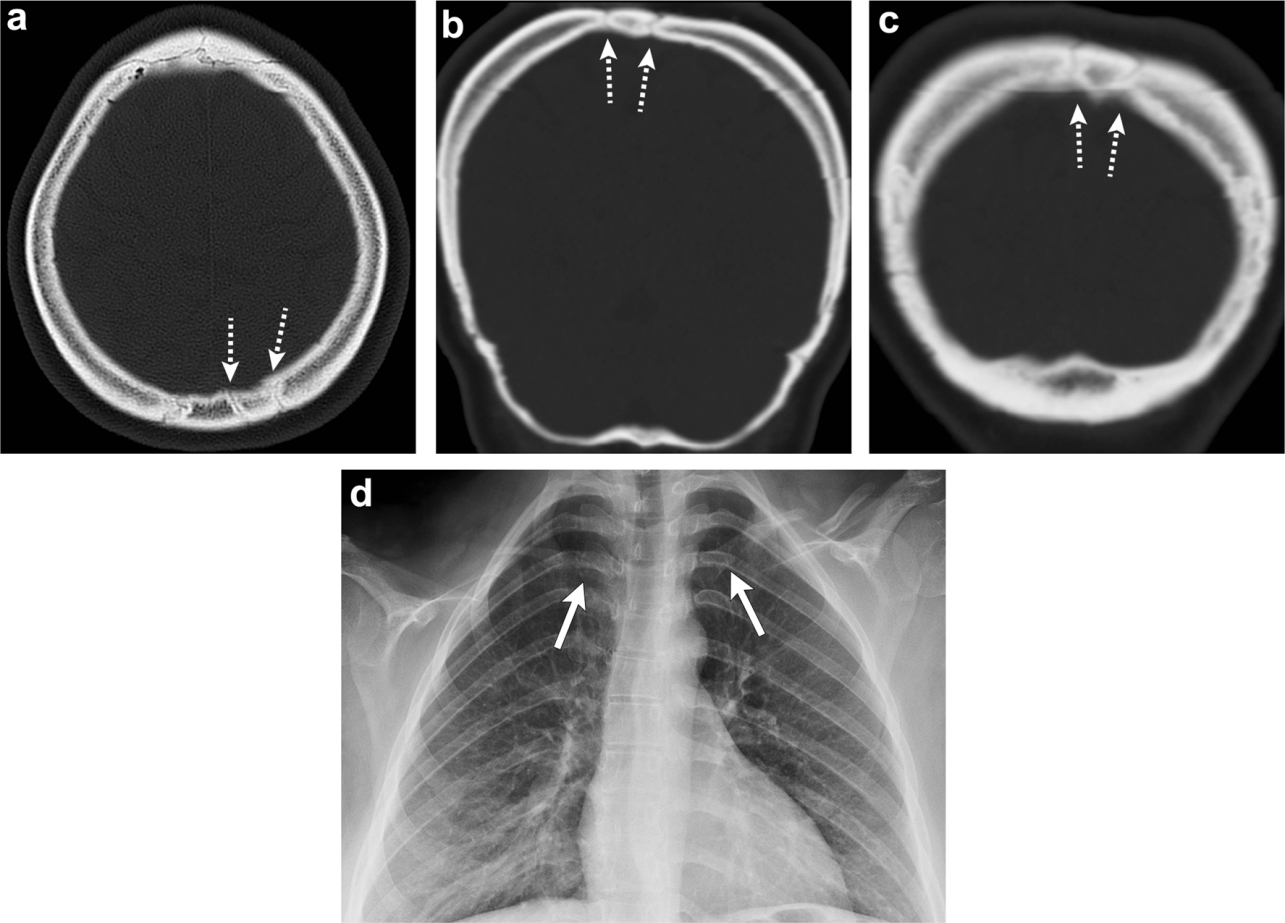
Cleidocranial dysostosis. Axial (a) and coronal (b, c) head CT images show islands of intra-sutural bones (*dashed arrows*) in the lambdoid and sagittal sutures representing wormian bones. Chest radiograph (d) in the same patient depicts hypoplasia of the bilateral clavicles (*arrows*), confirming cleidocranial dysostosis
